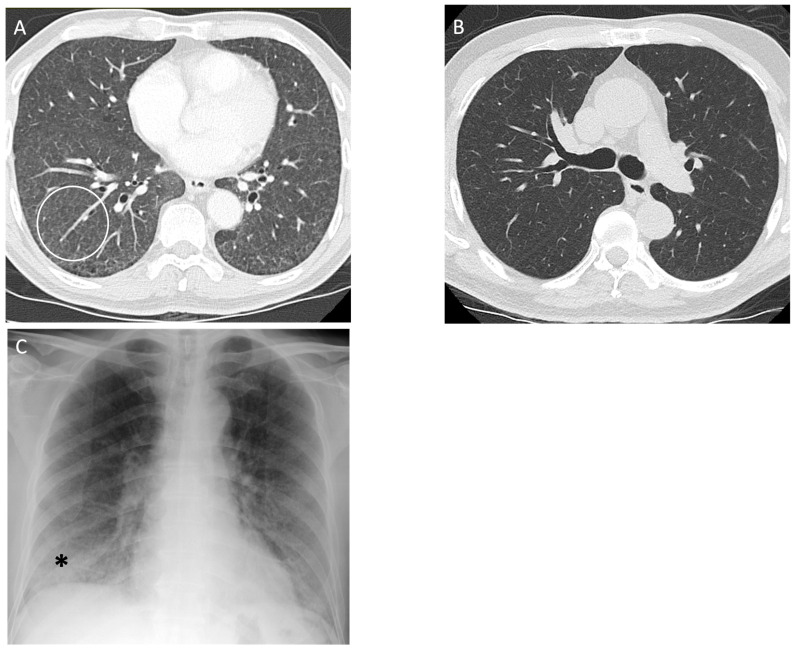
A 62-year-old non-smoking male with metal vapor exposure in the workplace was diagnosed with non-fibrotic hypersensitivity pneumonitis on surgical lung biopsy. (A) CT of lung bases demonstrates diffuse ground glass abnormality posteriorly (white circle); (B) follow up CT 6 months after being removed from the workplace demonstrates compete resolution of the opacities; (C) chest radiograph shows a subtle increase in lung density at the bases (asterisks) with relatively preserved lung volumes.
